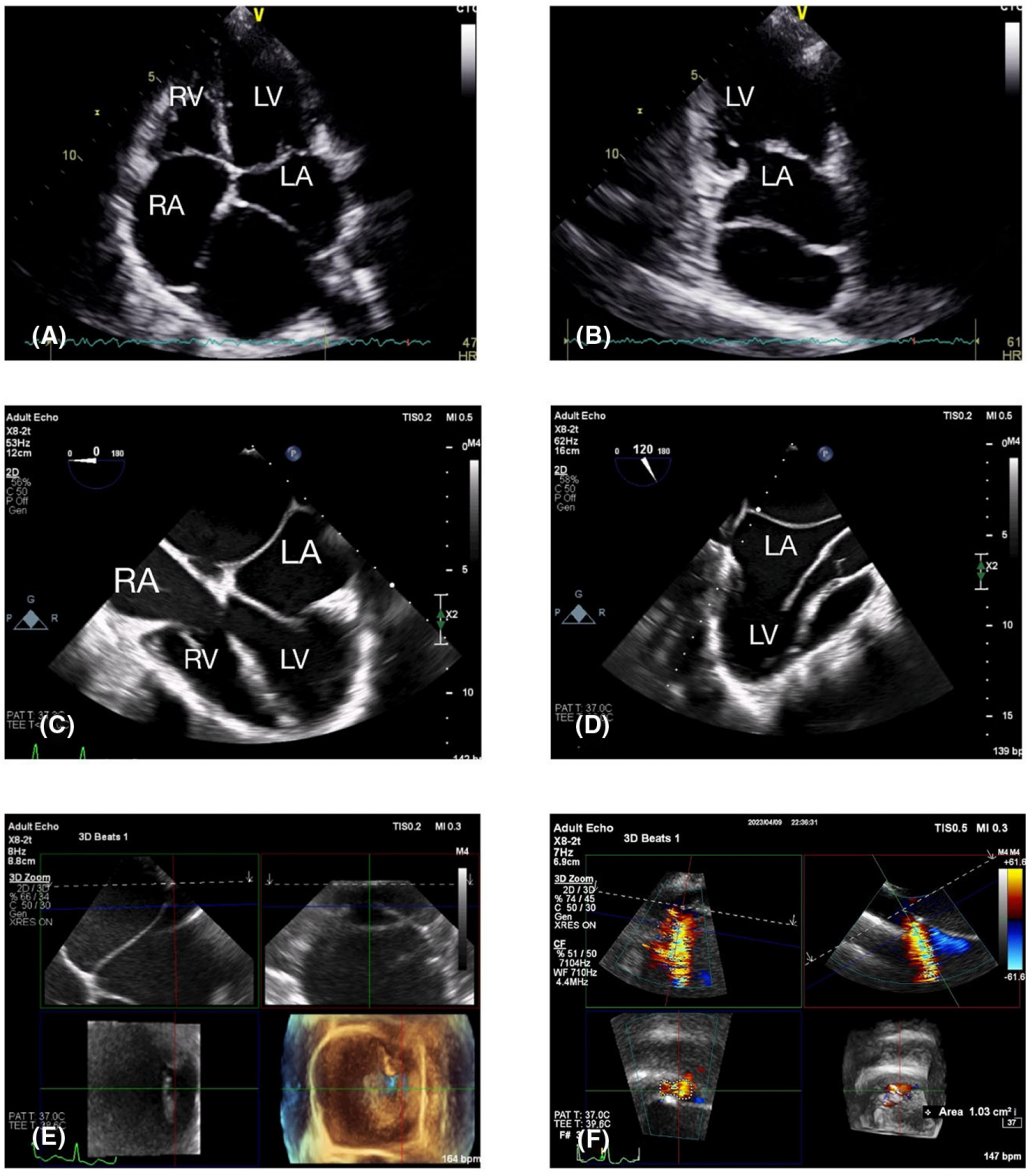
(A–F): Transthoracic and transesophageal echocardiography demonstrated an enlarged left atrium divided with a thin fibromuscular membrane into dorsal and ventral chambers (A–D), which communicate with each other with a small‐size fenestration measured 3 cm in diameter and having an area of 1.1 cm^2^ in 3D reconstruction (E, F).
